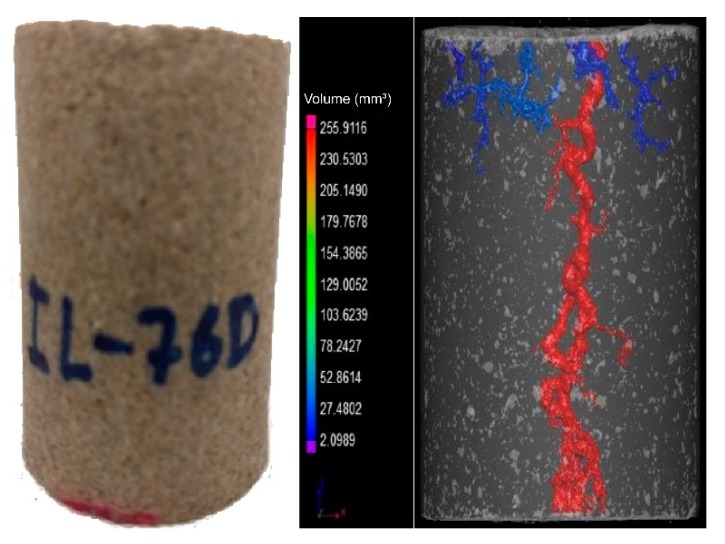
Imaging of wormhole structure using CT scan; ANS2; 0.5 mL/min (IL-76D).
